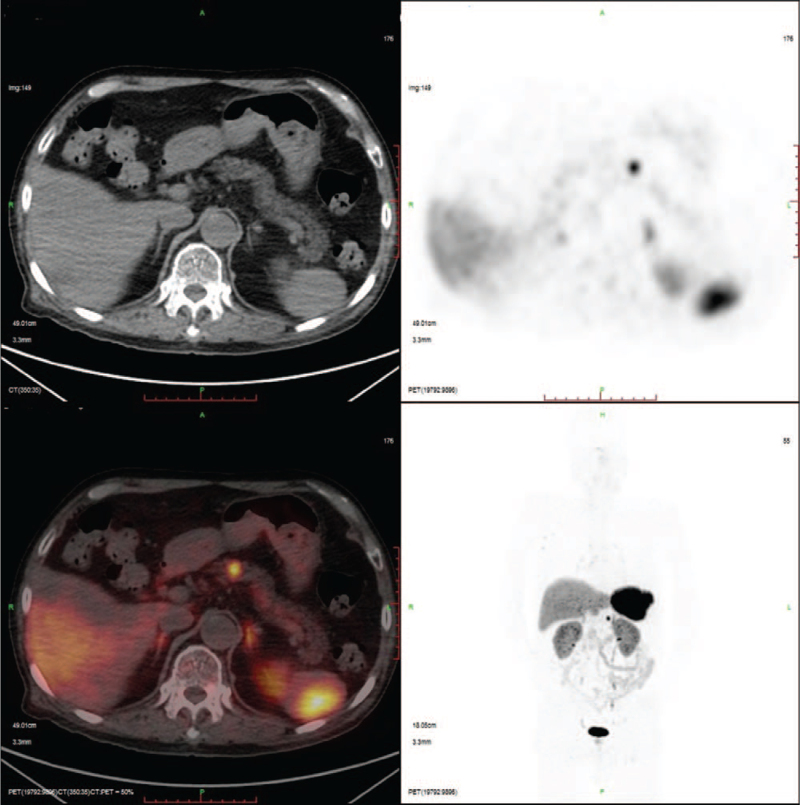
A lesion with high DOTATATE uptake approximately 1 cm in diameter in the pancreatic body showed by the ^68^Ga-DOTATATE PET-CT. ^68^Ga-DOTATATE = Gallium-68-labeled tetraazacyclododecanetetraacetic acid-Dphel-Tyr3-octreotate, PET-CT = Positron emission tomography-CT.
